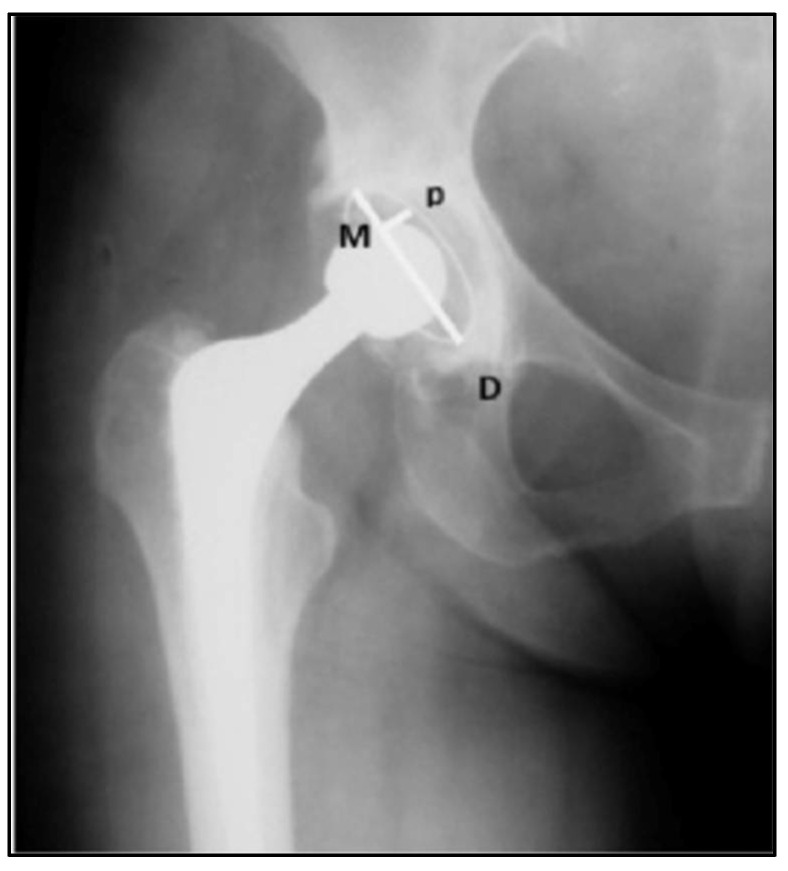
Radiographic areas of acetabular displacement, proposed by DeLee and Charnley, represented in the angle of lines M and D (1976) [43].
